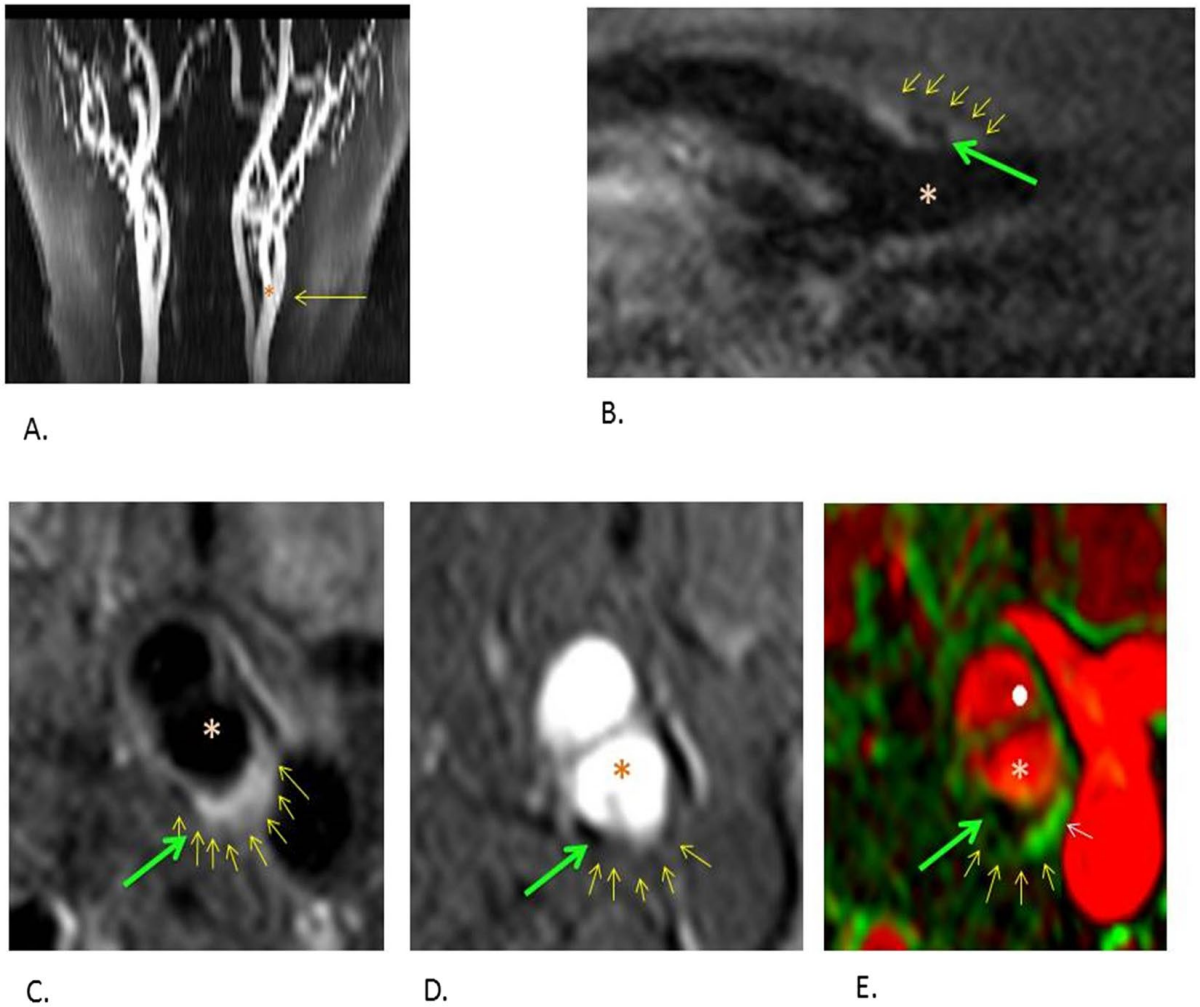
Representative images acquired during MRI. The small yellow arrows correspond to the outer border of plaque, the green arrows highlight an area of calcification within plaque and *highlights the lumen in each sequence. (A) A 2D time of flight with the yellow arrow pointing towards the bifurcation. (B) A black blood sagittal oblique section through the bifurcation. (C) A corresponding cross sectional black blood imaging sequence through the common carotid artery bifurcation where plaque can be seen at the posterior aspect of the vessel wall. (D) A corresponding bright blood image at the same level. (E) A DCE parameter map of the cross sectional image. Ktrans is estimated using a Patlak model and a Ktrans map is generated. The Ktrans signal is shown in green. The boundaries of the plaque defined on the T1 weighted sequence are applied to this map to estimate the Ktrans measurement within the plaque. The white arrow highlights the Ktrans signal within the plaque area.
